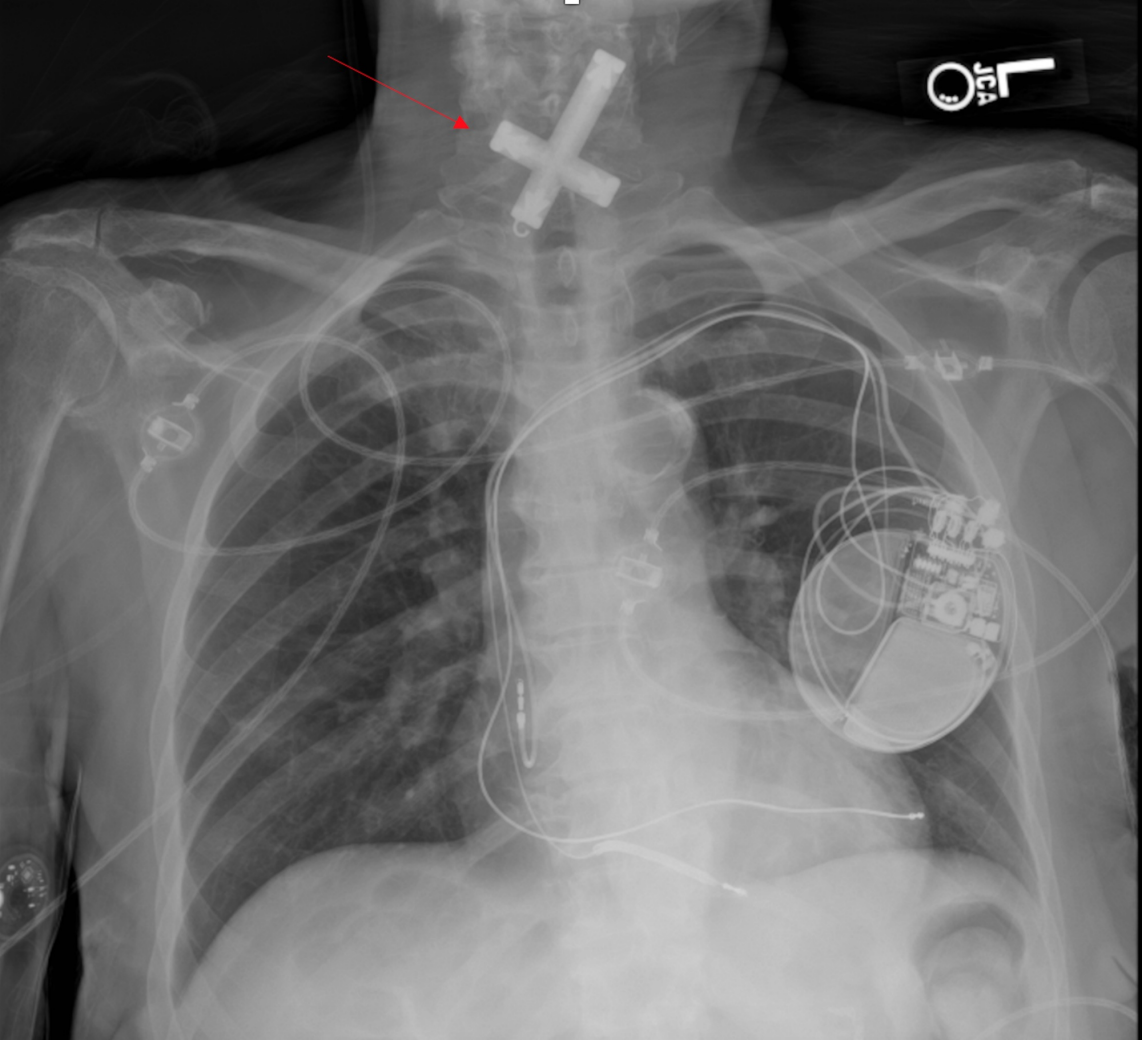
Posterioanterior chest radiography demonstrating a crucifix (red arrow) shaped object within the patient's neck.
